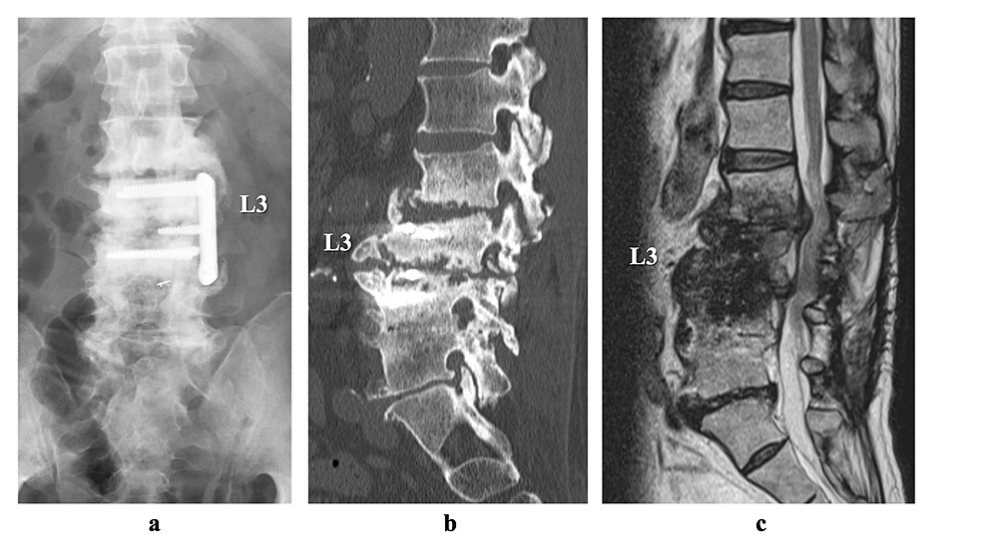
Images on admission. (a) Lumbar anterior-posterior plain radiograph of the lumbar spine showing the damaged L3/4 plate and L4 screw. (b) Computed tomography scan (sagittal slice) showing bone destruction of the vertebral endplates and osteosclerosis of the L2-4 vertebral bodies. (c) Sagittal T2-weighed magnetic resonance image showing the low-intensity signal of the L2-4 vertebral bodies.
